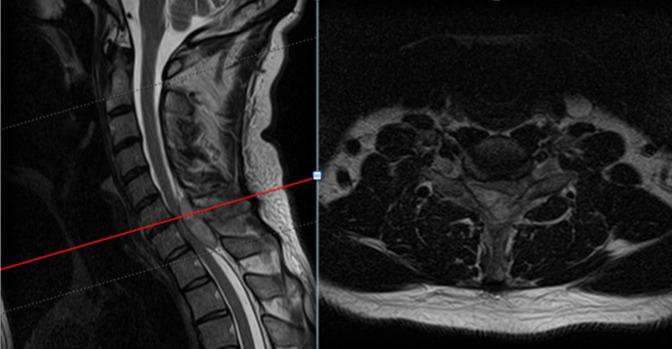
Sagittal and axial MRI images of the cervical spine at the C6–7 level demonstrating the posterior spinal lesion leading to spinal cord compression.
